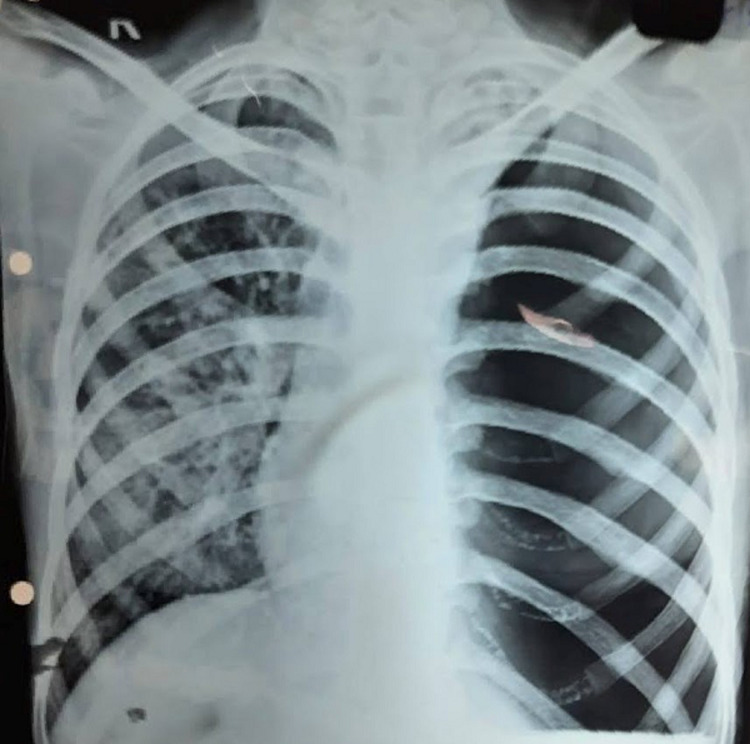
chest X-ray showing tension pneumothorax on left side
